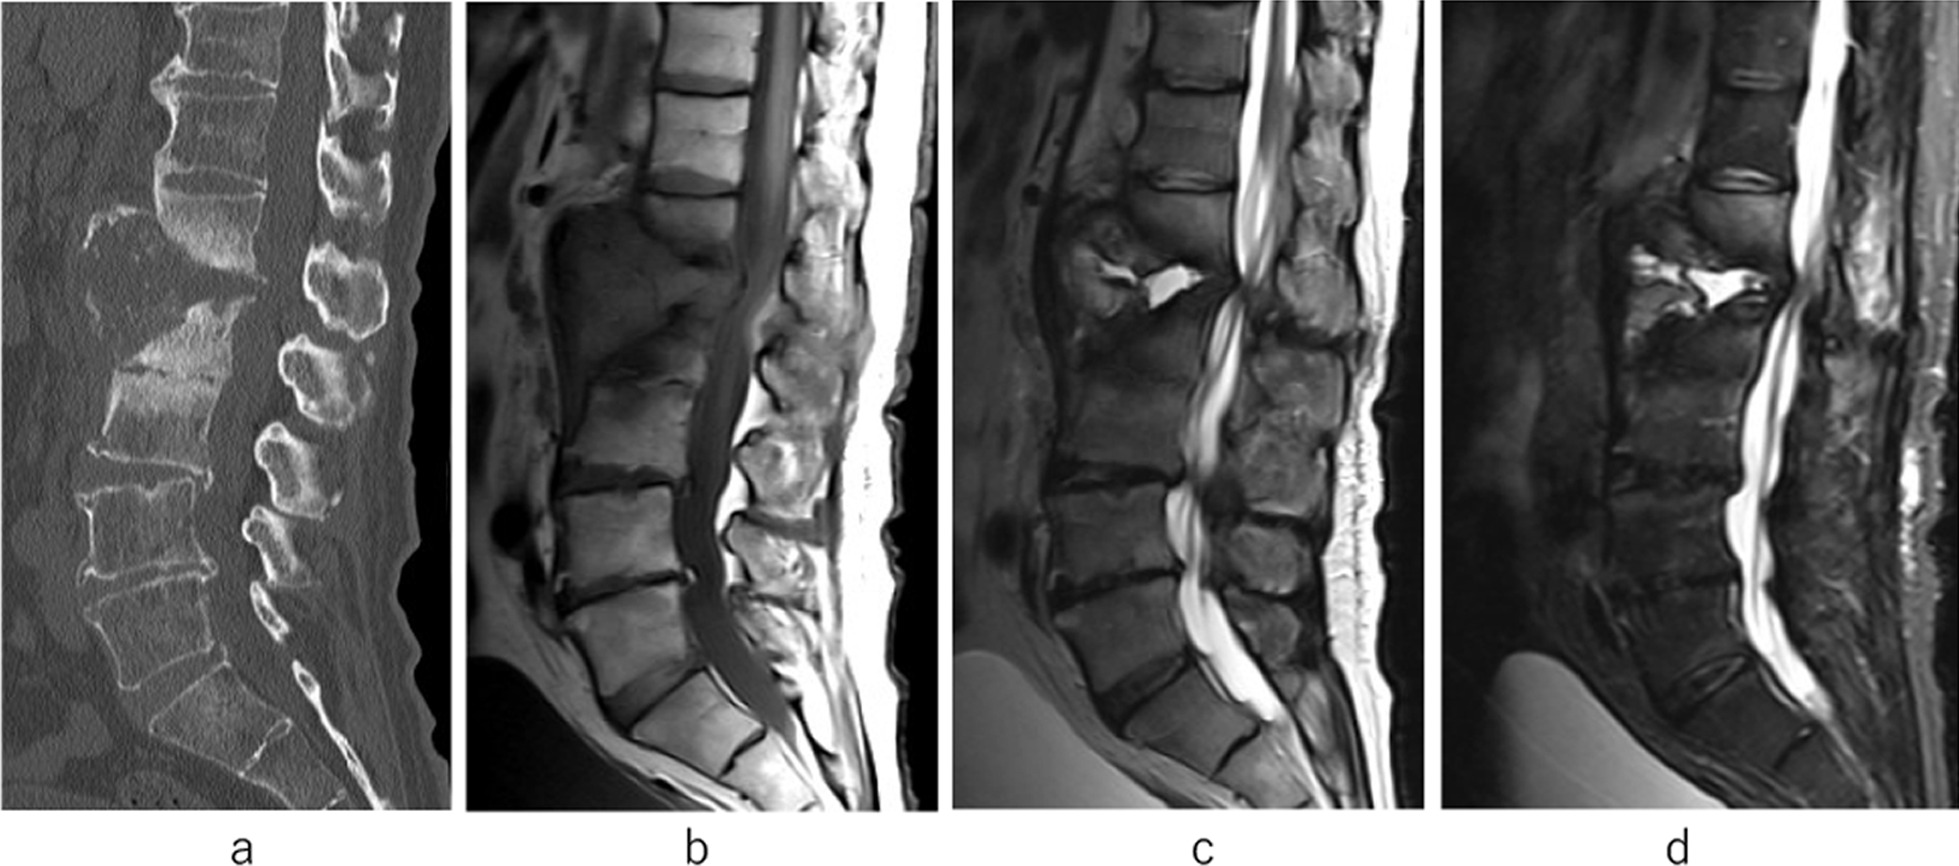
At the 4th hospitalization. A huge bone defect in L1 and L2 is shown on computed tomography (a). MRI shows an abscess in the L1–2 disc space that was enlarged toward the anterior (b–d)
